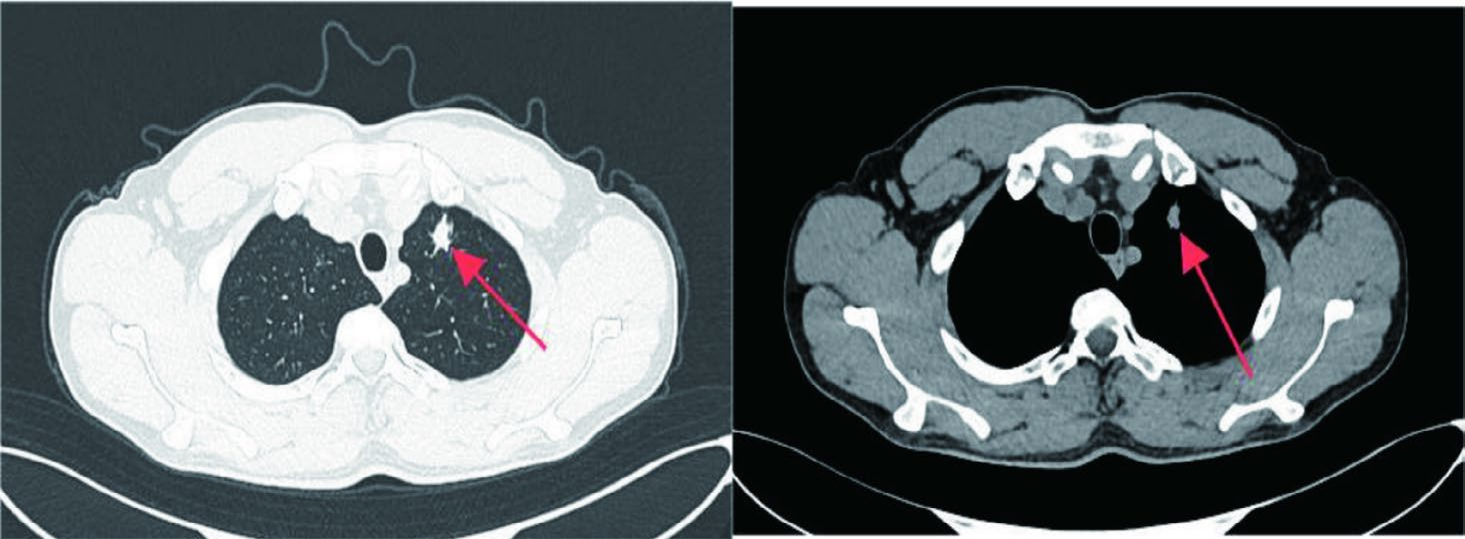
The patient's chest CT scan revealed a solid nodule (red arrow) at the apicoposterior segment of the upper left lobe of the lung, located close to the pleura, measuring approximately 1.8 × 1.2 cm. There are visible spiculated signs around the nodule, and no calcification was observed on the mediastinal window.
